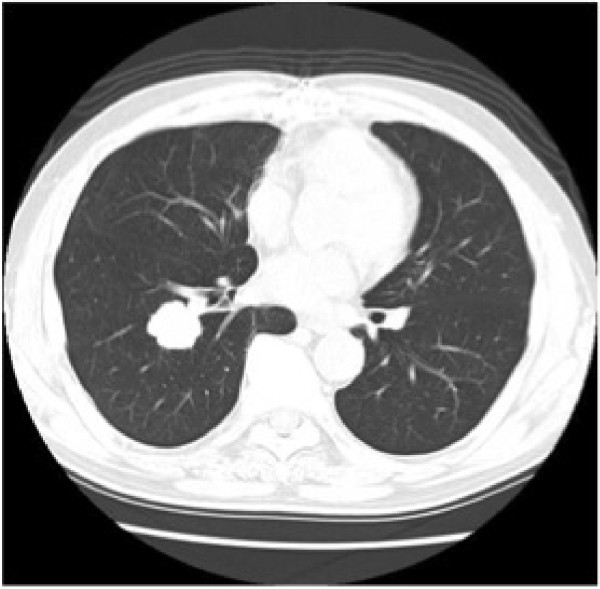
Another computed tomography (CT) scan shows a 2.5 cm well-defined nodule in the right lower lobe at seven years.
